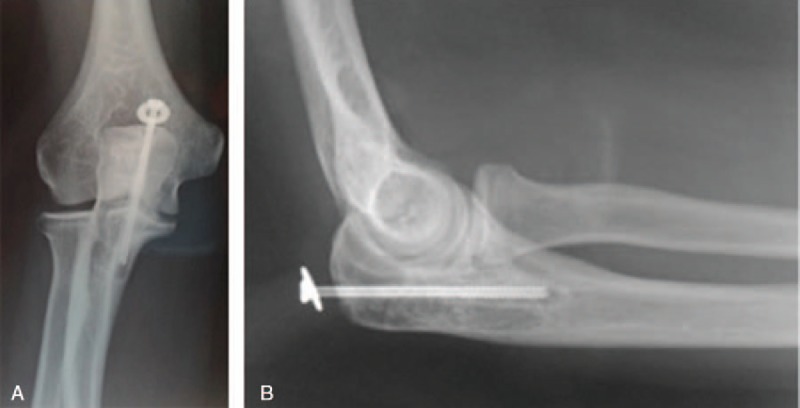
Implant loosening in a male patient (15 years old) treated 60 days before with a single cannulated screw. X-rays in anterior–posterior (A) and lateral (B) planes.
